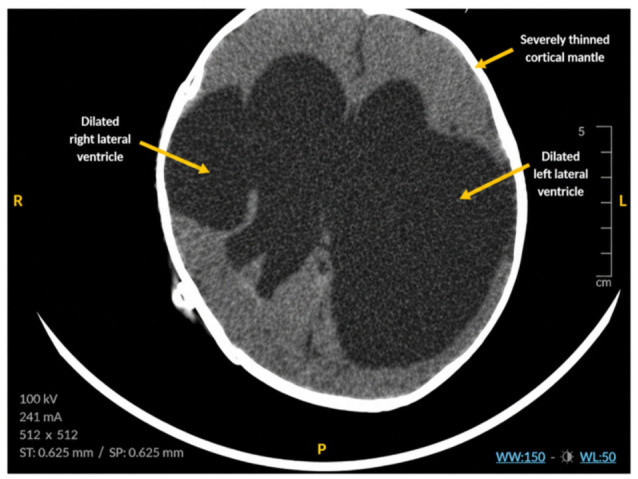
Axial non-contrast CT demonstrating marked dilatation of the lateral ventricles with severe cortical mantle thinning, consistent with advanced ventriculomegaly. R = right; L = left; P = posterior.
